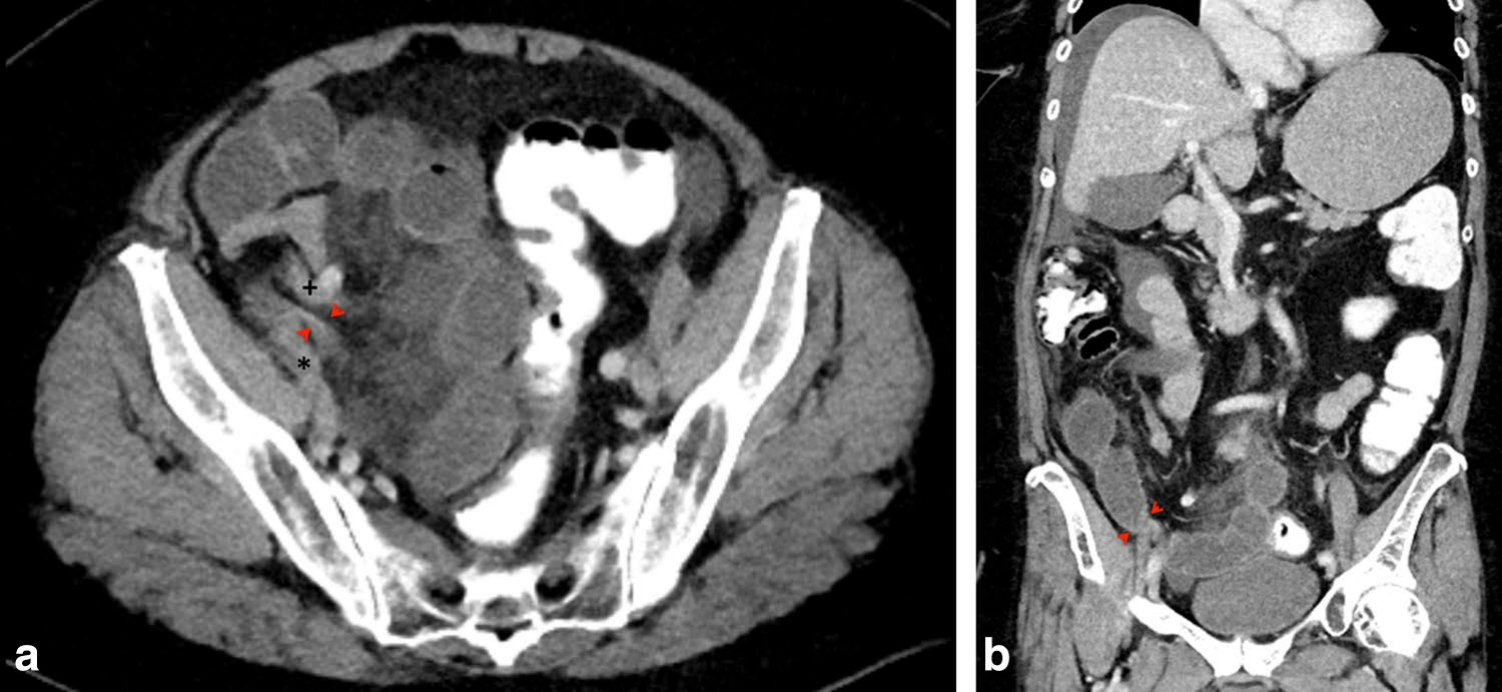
CT scan. a Axial view showing herniation of ileum with abrupt reduction of caliber (arrowheads) between external iliac artery ( +) and external iliac vein (*). b Coronal view, showing the site of herniation (arrowheads). Distension of proximal jejunal loops due to small bowel obstruction. Perihepatic and inter-enteric ascites in the right hemiabdomen as secondary finding
